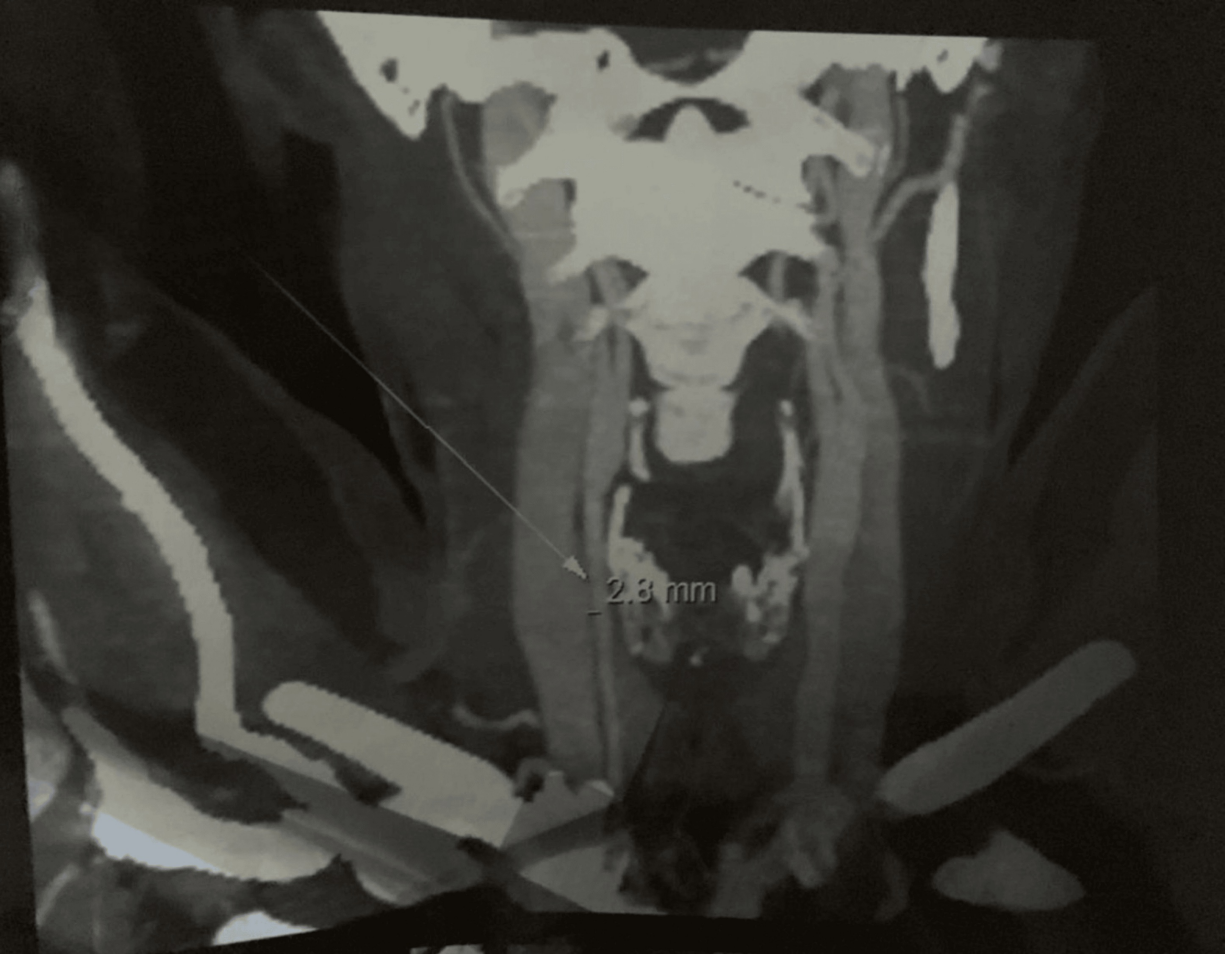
Left common carotid artery: focal wall thickening. Axial CT angiography of the left common carotid artery demonstrates focal mural thickening with a measured wall thickness of 2.8 mm. The arrow indicates the site of measurement along the arterial wall.
